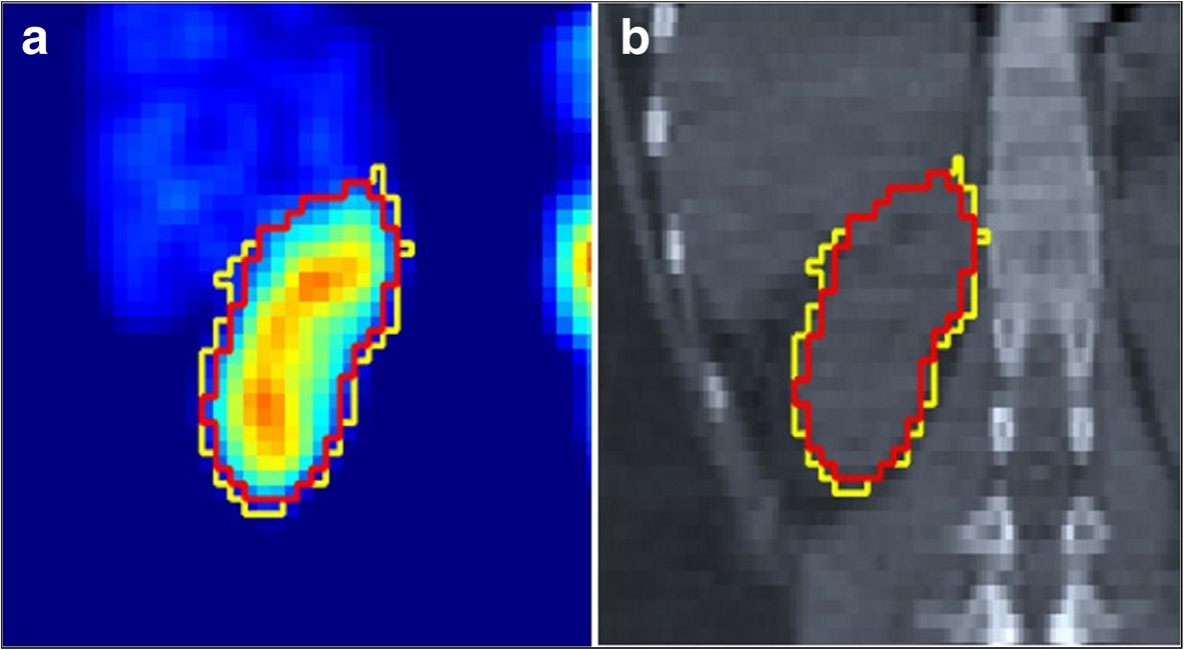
An example of the right kidney segmentation based on dual iterative thresholding method in SPECT (a) and CT (b). The activity threshold was set to 25% (yellow line), and the volume threshold was equal to 36% (red line)
